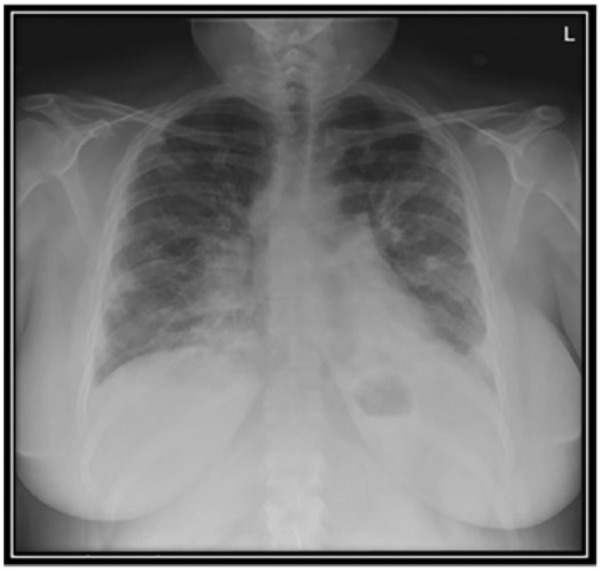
Chest X-ray: acute onset of diffuse bilateral alveolar and interstitial opacities.
